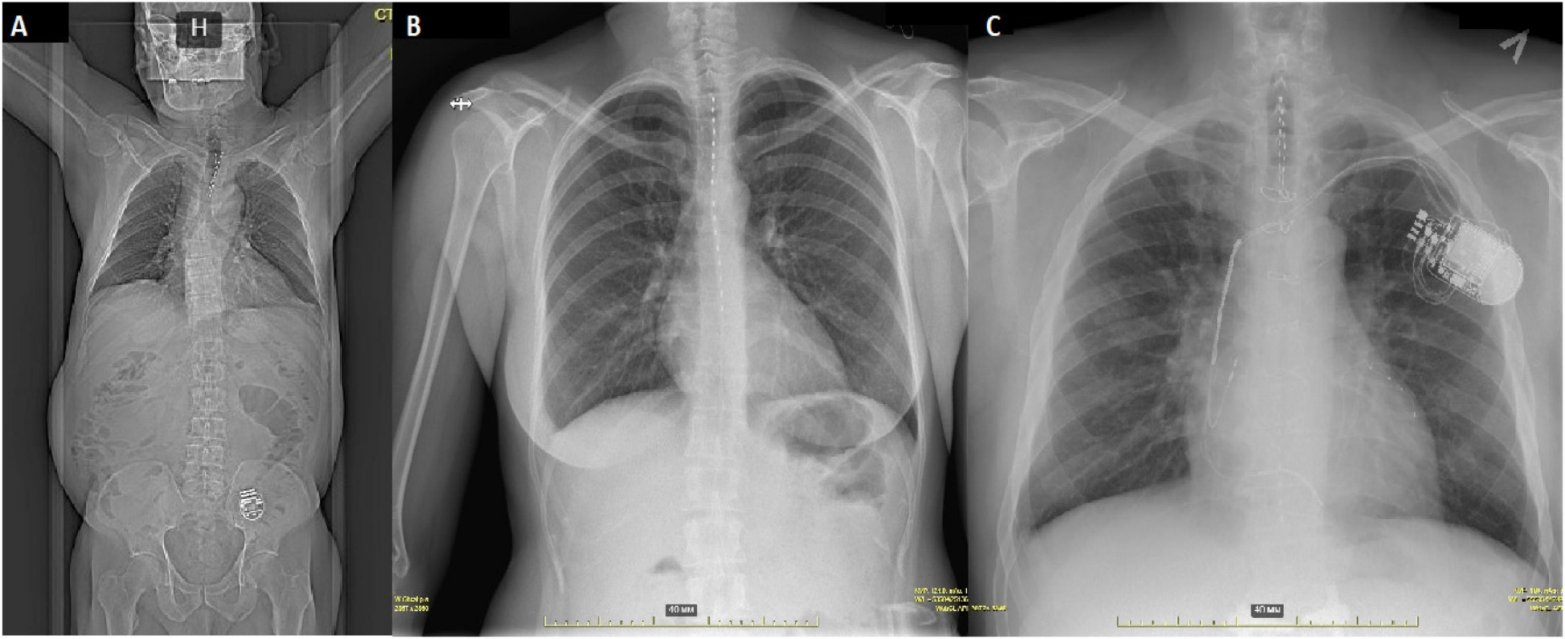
Radiographic imaging in different clinical cases. (A) Computed tomography showing normal electrode and pulse generator positioning. (B) Sequential electrode placement in a patient with generalized artery spasms, including coronary and celiac artery. (C) Spinal cord stimulation in a patient with a CRT-D device and an implanted stimulator for persistent spinal pain syndrome type 2.
